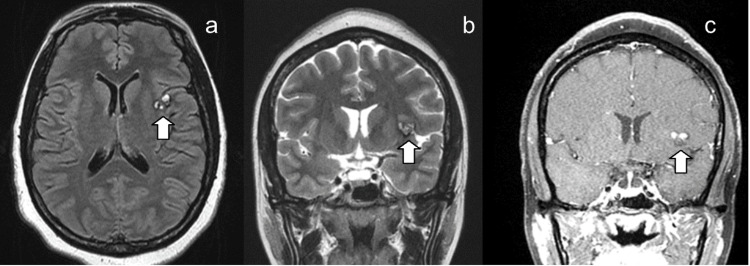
Magnetic Resonance Imaging (MRI) of the Head With/Without Contrast Impression: Area of susceptibility noted adjacent to the left insular cortex with parenchymal and subarachnoid components correlating with the hyperdensity as seen on prior CT head, consistent with hemorrhage (white arrow). (a) Axial view; (b) Coronal view; (b) Coronal view post-contrast.
